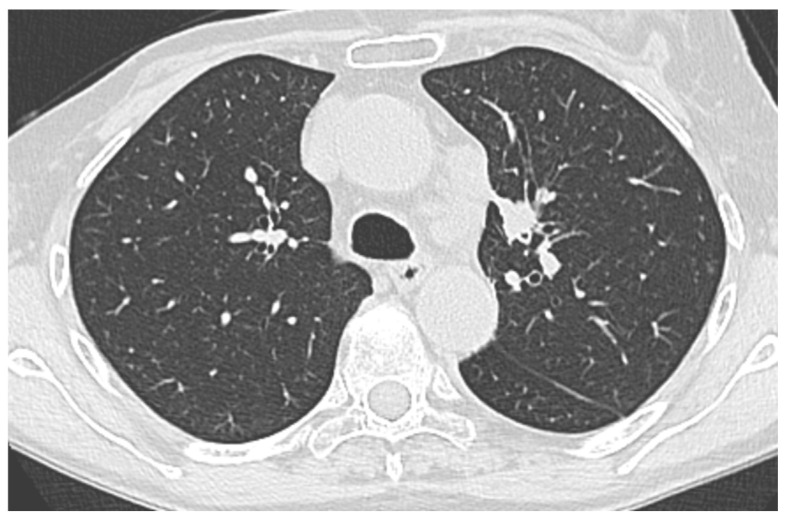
Chest CT scan example of a peripheral pulmonary lesion (PPL) sampled with a thin bronchoscope (TB).
